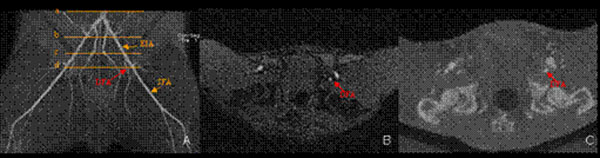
A maximum intensity projection TOF image (A) shows the vessel areas measurement at different level. The four orange lines (a-d) denote the position located at distal aorta (a), the proximal (b) and distal external iliac artery (EIA) (c), and the proximal deep femoral artery (DFA) (d), respectively. The left DFA (red arrow) is demonstrated at an axial image on TOF MRA and CTA (B-C).
